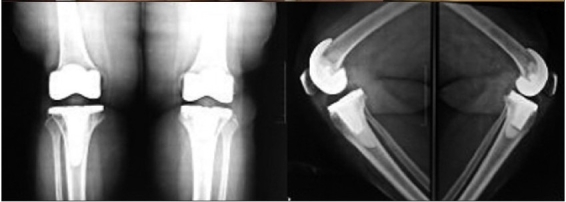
Bilateral anteroposterior and lateral radiographs at 18 month, follow-up of the same patient
